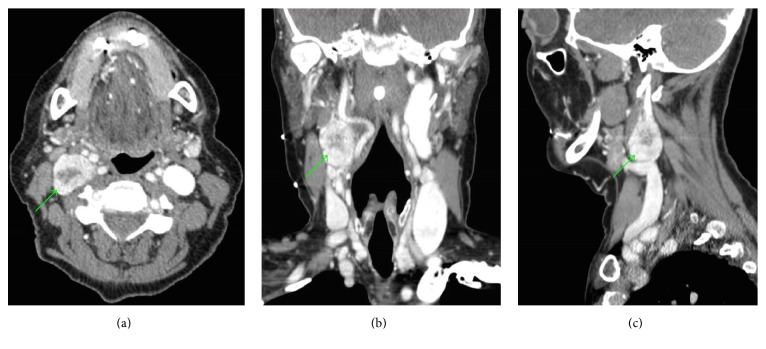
CT angiography of the neck with axial (a), coronal (b), and sagittal (c) sections of the heterogeneously enhanced irregular 2.4 × 2.2 × 2.2 cm mass within the right carotid sheath. The green arrows refer to the neck mass.
